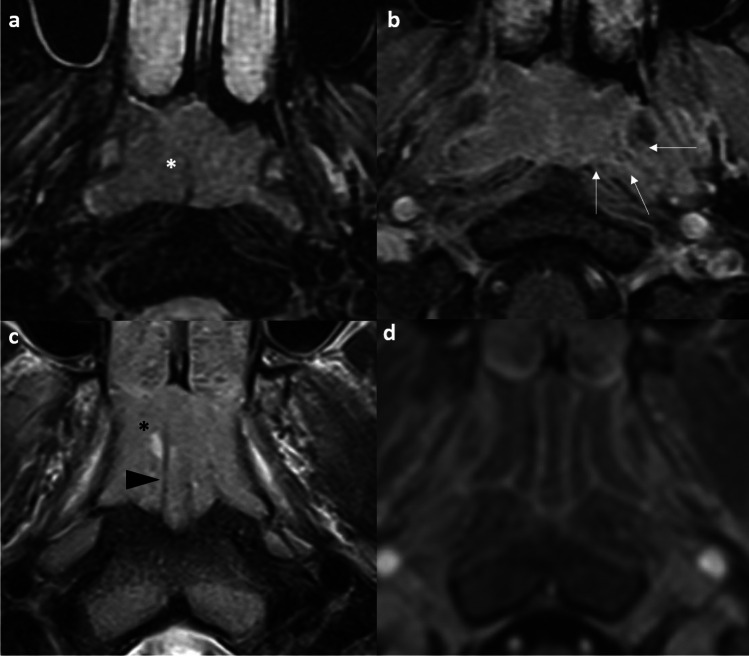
Distinction between nasopharyngeal tumor (a & b) and adenoidal hyperplasia (c & d). Axial T2-weighted, fat-suppressed image shows intermediate signal slightly asymmetrical soft tissue occupying the bilateral nasopharynx with absence of the adenoidal septa (asterisk) (a). Axial T1-weighted post-contrast image with fat suppression shows moderate enhancement of the bilateral nasopharyngeal soft tissue mass with focal loss of the deep white mucosal line in the left lateral and posterior wall (arrows) (b). Biopsy confirmed nasopharyngeal carcinoma. Axial T2-weighted, fat suppressed image shows the symmetrical soft tissue in the bilateral nasopharynx containing cystic focus (asterisk) and preservation of hypointense adenoidal septa (arrowhead) (c). Axial T1-weighted post-contrast image with fat suppression shows symmetrical hypo-enhancing soft tissue with a striated appearance and preservation of deep mucosal white line (d). Findings are consistent with adenoidal hyperplasia
